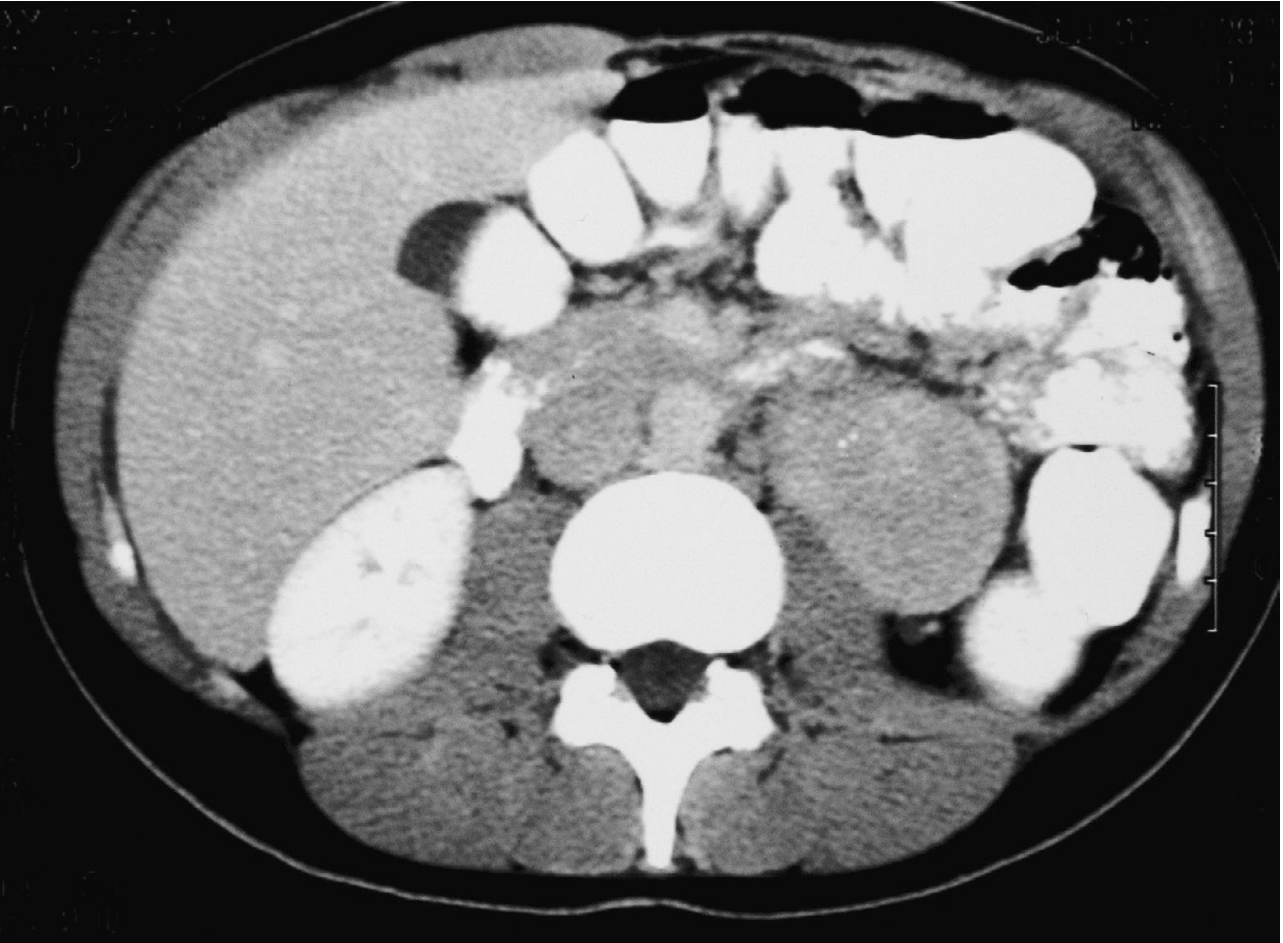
Computed tomography scanner showing a large left-pararenal mass (Hyalin-vascular type of Castleman's disease). Note the presence of microcalcification (white spot) within the mass.
